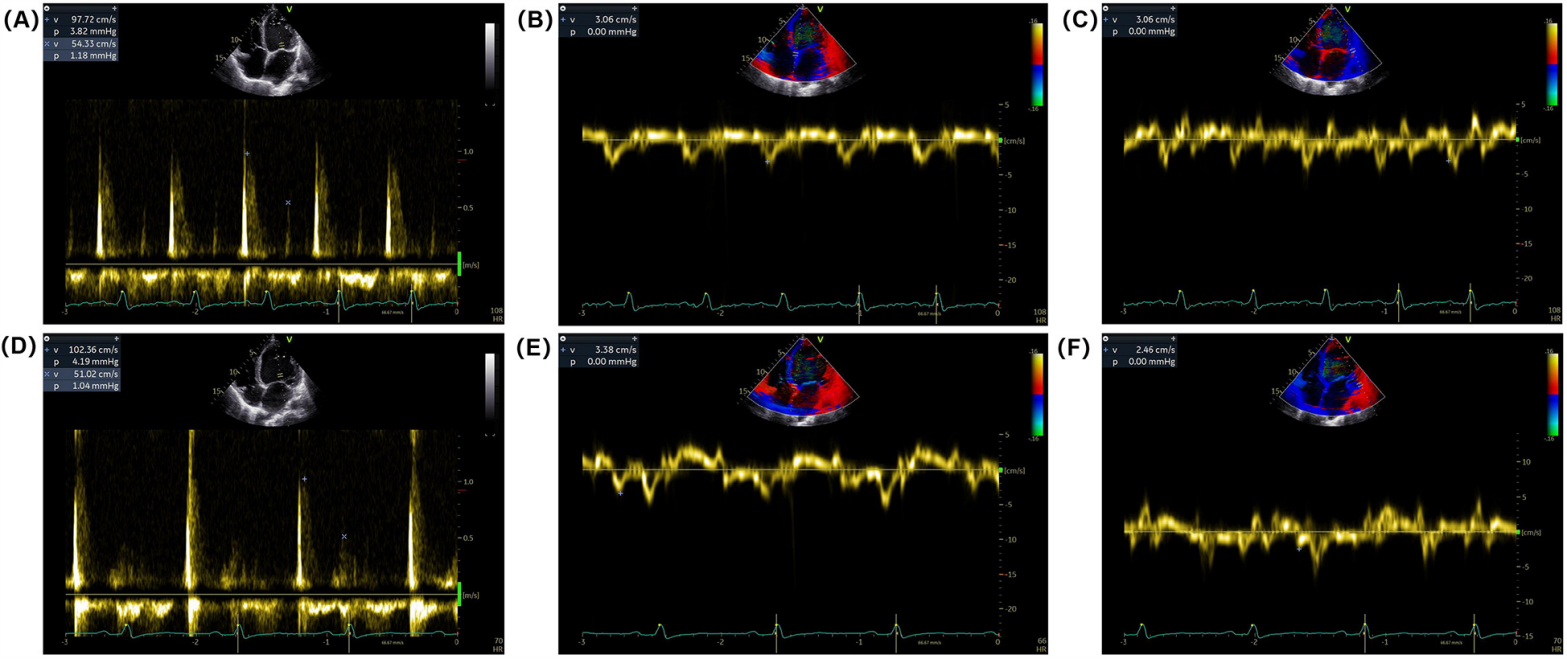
Mitral inflow and tissue Doppler imaging for diastolic function assessment. (A) Pulsed-wave Doppler recordings of mitral inflow showing (E,A) waves at admission. (B,C) Tissue Doppler imaging of the mitral annulus showing early diastolic velocity (e’) at the septal and lateral annulus at admission. (D) Pulsed-wave Doppler recordings of mitral inflow showing (E,A) waves at discharge. (E,F) Tissue Doppler imaging of the mitral annulus showing early diastolic velocity (e’) at the septal and/or lateral annulus at discharge.
